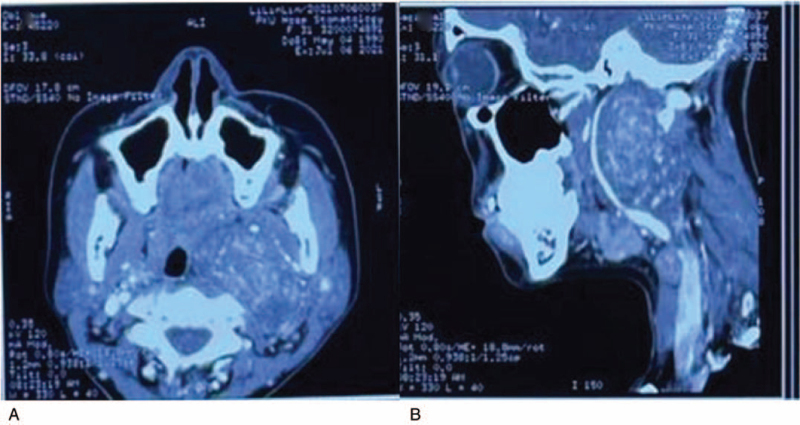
(A,B) CT showed shadows of a 6.8 × 5.4 × 3.4 cm soft tissue masses with uneven reinforcement density in the left neck and parapharyngeal area. The superior boundary of the shadows reached the skull base, the inferior boundary reached the level of the hyoid bone, and the boundaries were clear. There were speckled calcifications and thickened and tortuous blood vessels in the image. The left pharyngeal cavity was compressed and narrowed. CT = computed tomography.
